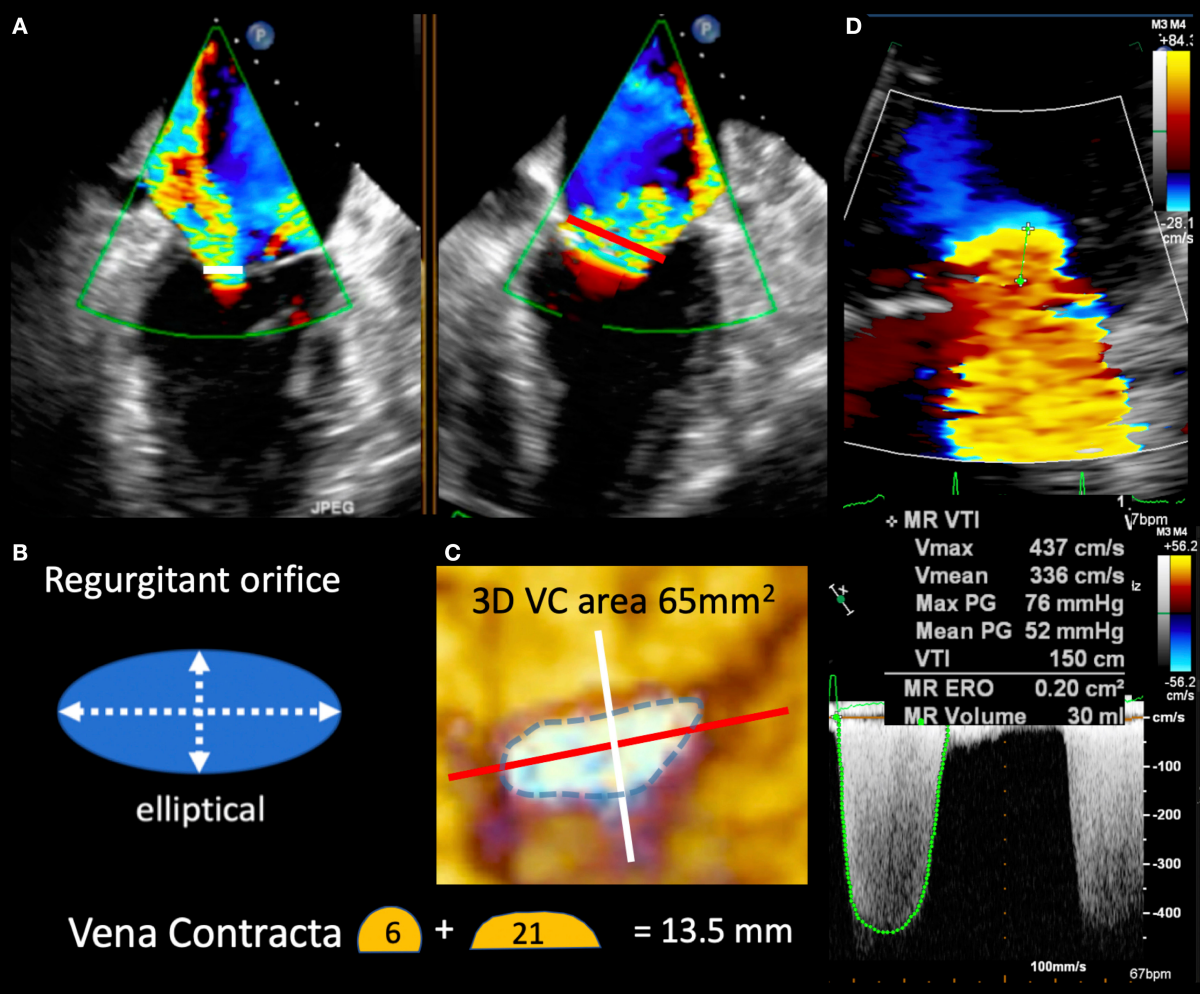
TEE image in “disproportionate” secondary MR demonstrating the vena contracta in two planes (white and red lines) seen to be longer in the commissural view. (B,C) Depict the regurgitant orifice morphology as elliptical and explain the vena contract size differences seen in (A). 3D vena contracta area of 65 mm^2^ better reflects the large elliptic regurgitant orifice compared with EROA of 20 mm^2^ by standard 2D Doppler method seen in (D).
